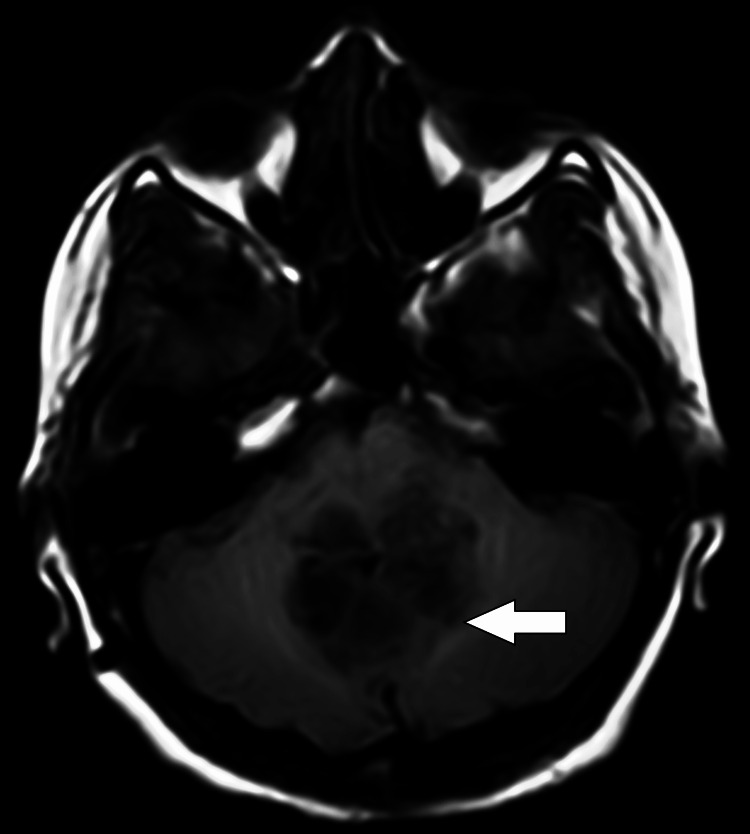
Axial T1 weighted brain MRI The white arrow shows the lesion appears predominantly hypo-intense. MRI: Magnetic resonance imaging
